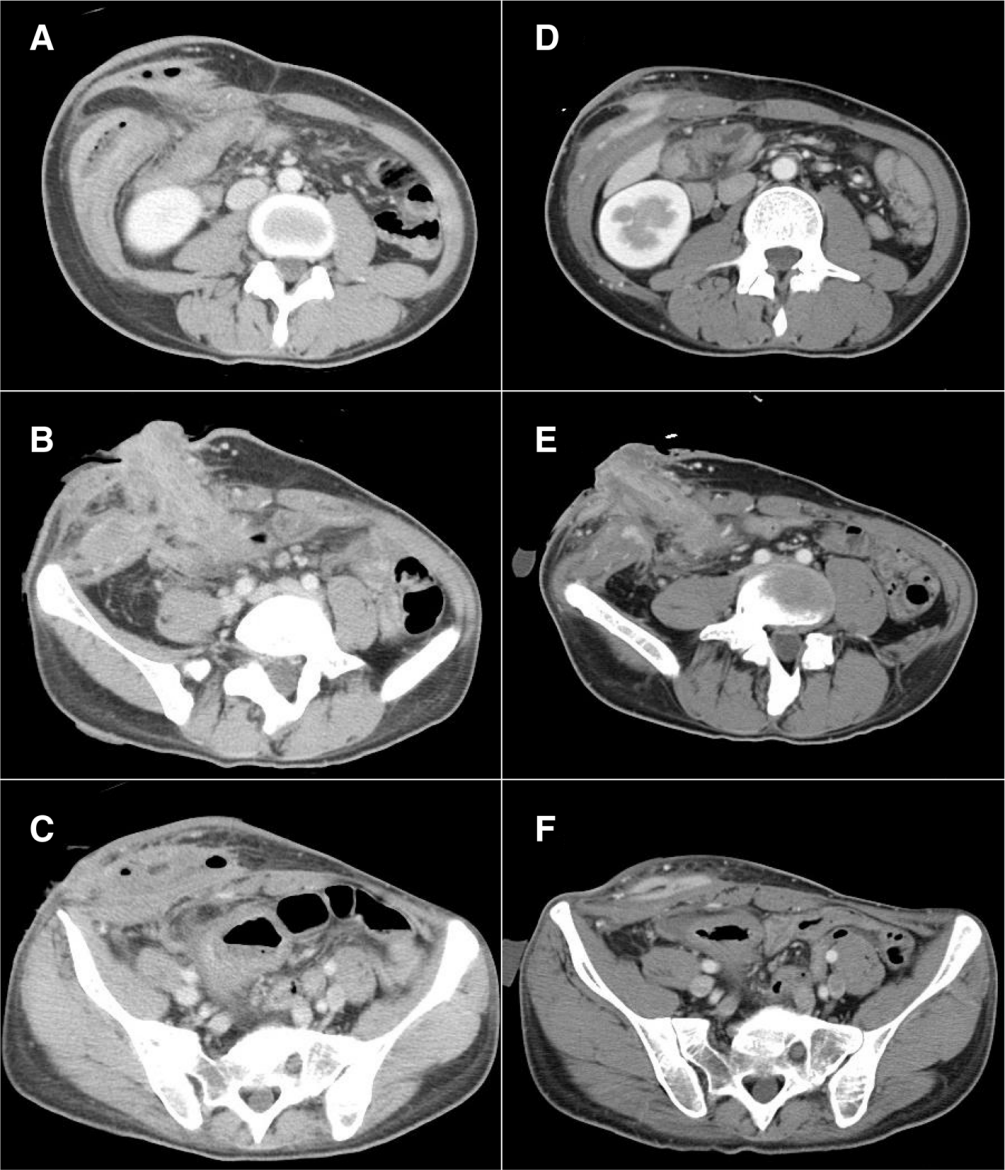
Representative abdominal contrast-enhanced CT scans. a–c Images obtained 3 weeks before surgery. Abscess cavities have formed in the abdominal wall and inflammatory changes are visible around the stoma site. d–f Images of CT scans obtained 1 week before surgery. The inflammatory changes have improved considerably, but the abscess cavities remain
